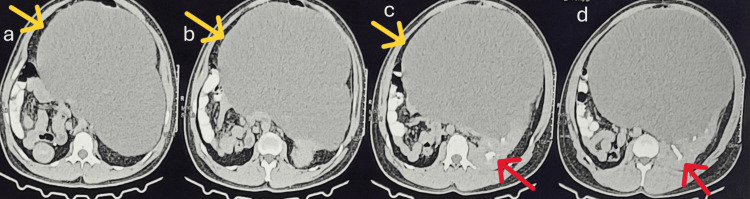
Axial contrast-enhanced CT images. (a-c) Extension (marked by yellow arrows) of the parapelvic cyst to the contralateral side of the abdomen. (c) Contrast opacification (marked by a red arrow) of the pelvicalyceal system. (d) The proximity of the cyst wall to the pelvicalyceal system (marked by a red arrow) shows no contrast within the cyst cavity, thereby confirming the non-communicating nature of the cyst.
